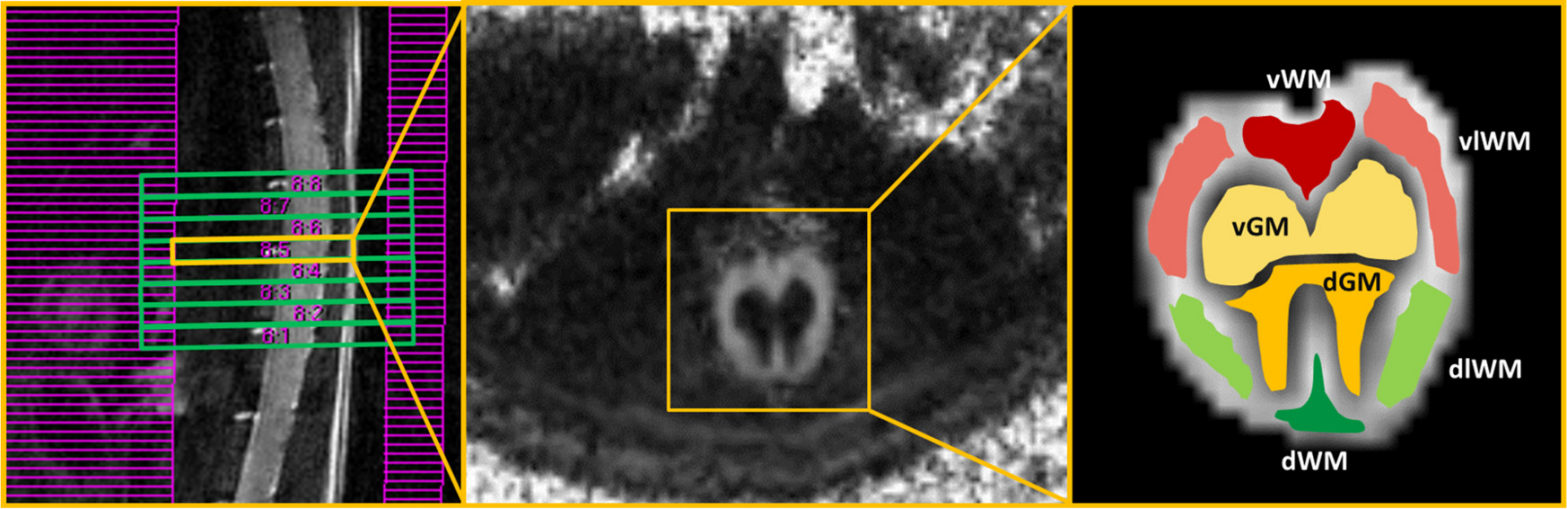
Acquisition setup and ROI delineation. Left: T2-weighted sagittal view of the lumbar tract. The green boxes are the image slices and the purple grid shows the position of the saturation slices. Note that the examined lumbar spinal cord region is mostly straight and the imaging slices were placed perpendicular to the axis of the spine. Center: FA map of a single slice (framed in yellow on the left panel). Right: ROIs overlaid on a part of the FA map including the whole section of the spinal cord (in yellow on the central panel); ventral (vWM), dorsal (dWM), ventro-lateral (vlWM) and dorso-lateral (dlWM) white matter, ventral (vGM) and dorsal (dGM) gray matter.
